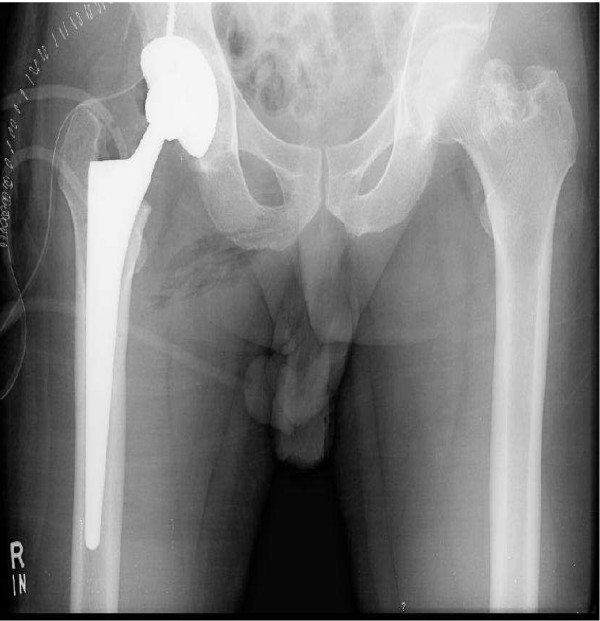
Showing postoperative radiograph after total hip arthroplasty.
